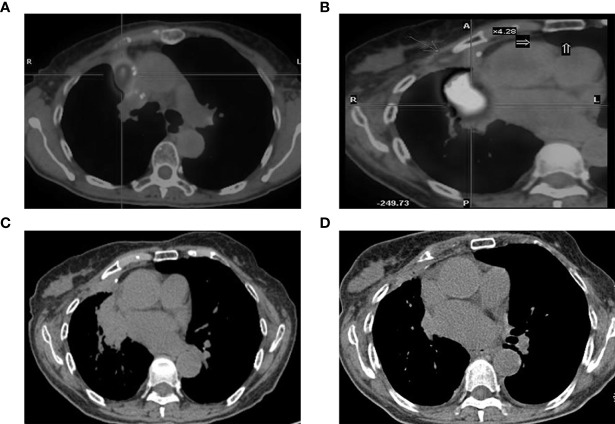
CT findings. (A) tumor status at diagnosis; (B) the increased tumor progress; (C) chest CT scan result before the afatinib treatments and (D) the chest CT scan result after two months of afatinib treatments. CT, computed tomography.
